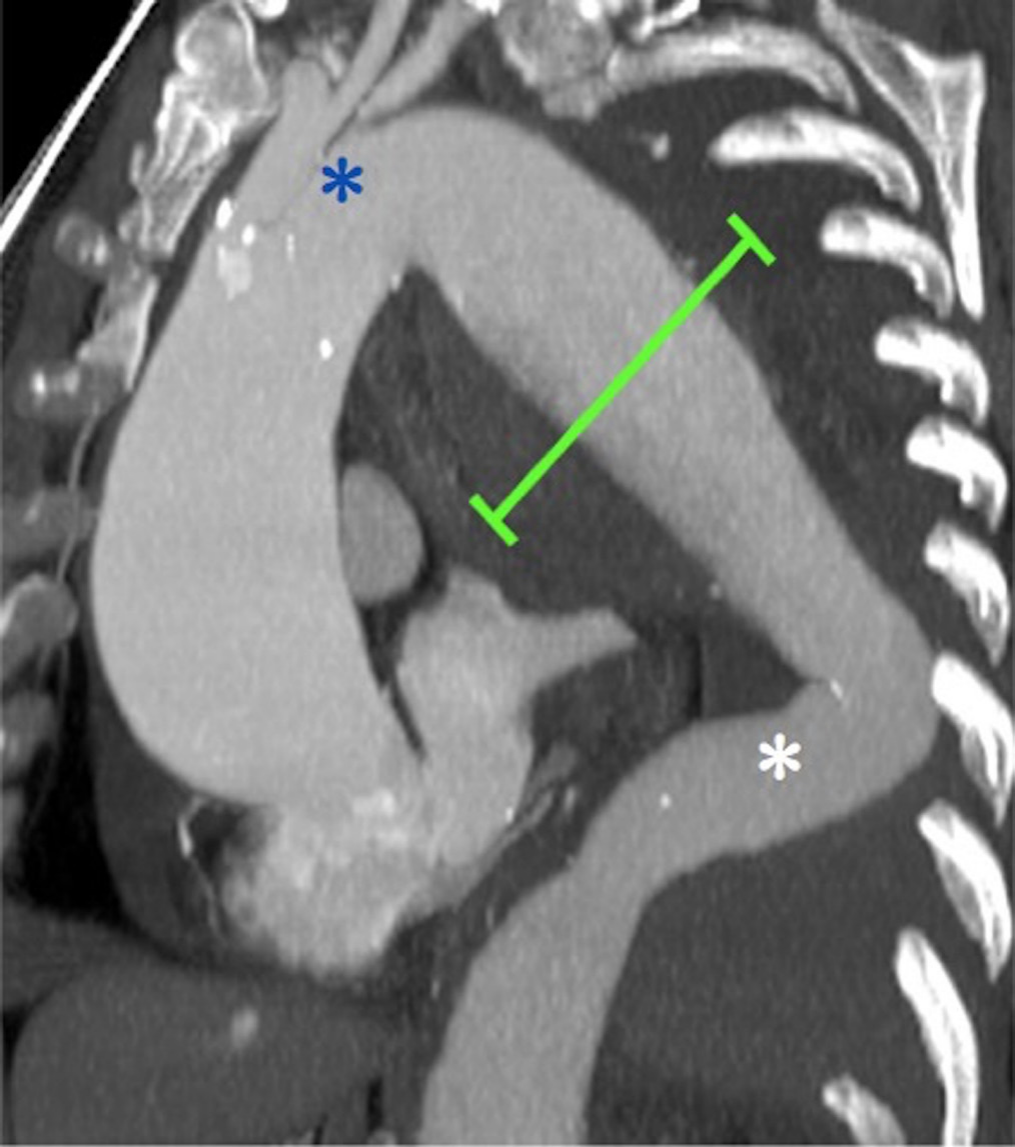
Preoperative CT MultiPlanar Reconstruction showing a 9-cm (line) ruptured descending thoracic aortic aneurysm with short and angulated proximal landing zone (upper asterisk) and an unclear and angulated distal landing zone (lower asterisk).
